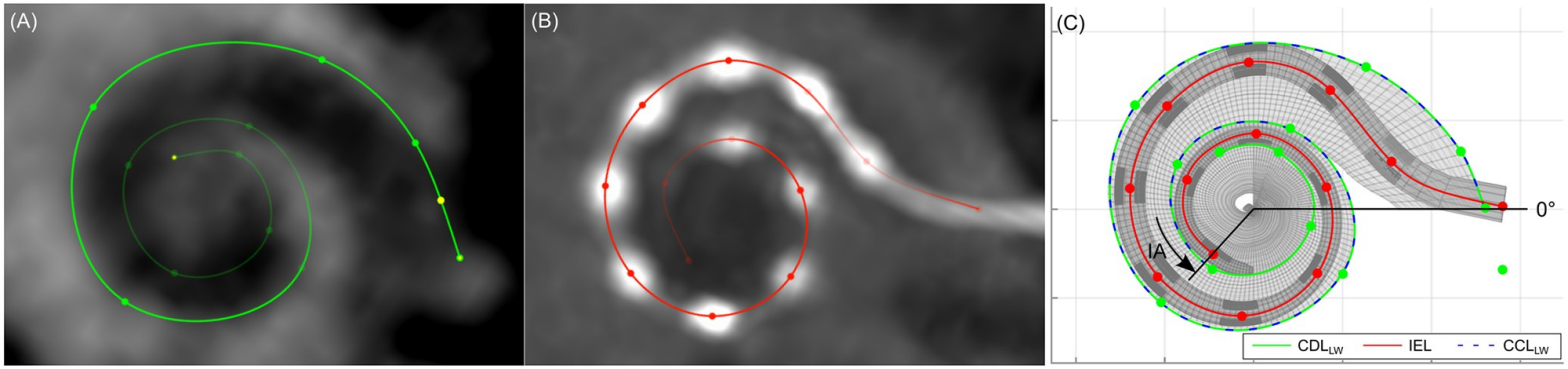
Visualization of the imaging data analysis method. First, pre-operative CBCT scans are used to trace the lateral wall of the cochlea (A). Then, post-operative CBCT scans are used to trace the electrode array by marking the position of each electrode contact (B). Finally, the two traces are combined to obtain a reconstruction of the implanted cochlea that can be used to derive anatomical and positional parameters (C).
